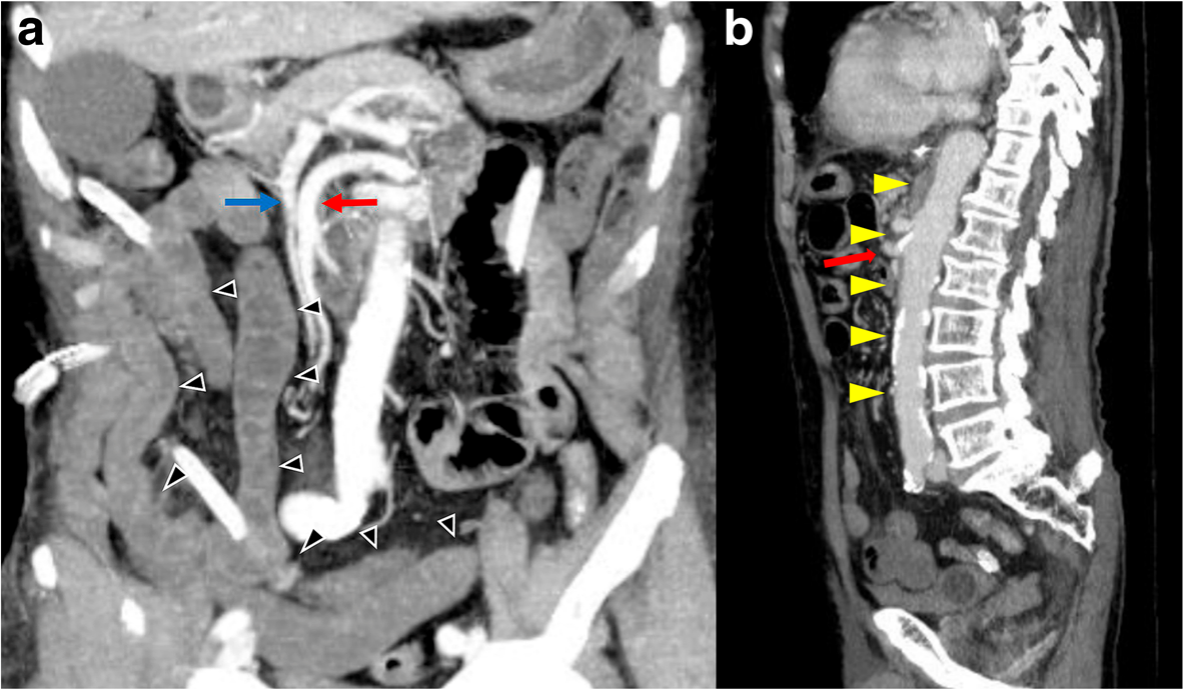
a, b Follow-up CT indicated an AD (yellow arrowheads), smaller SMV sign, and segmental lack of enhancement in the intestinal wall (arrowheads). SMV (blue arrow) was smaller than SMA (red arrow)
